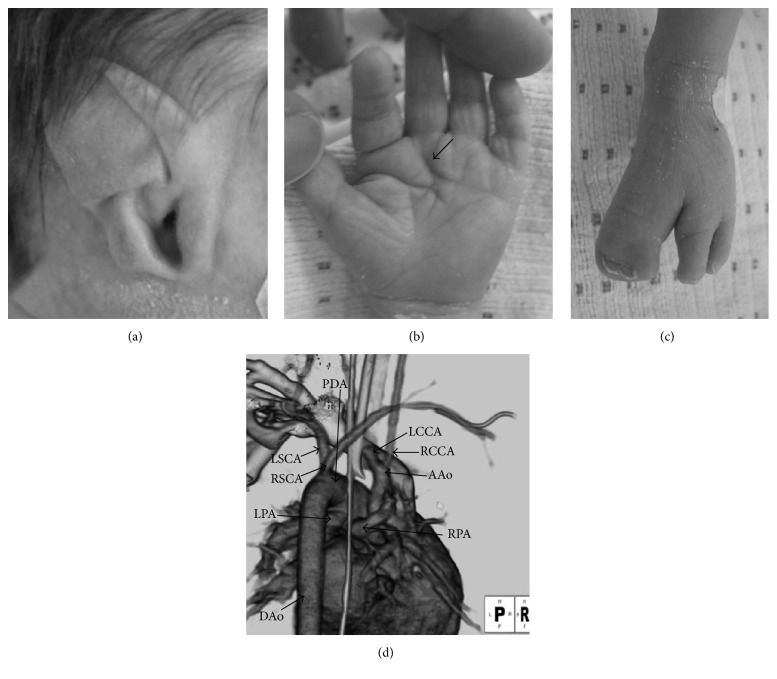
Dysmorphic features and cardiovascular malformations in Case 1. (a) “CHARGE ear.” (b) Hockey-stick palmar crease (arrow). (c) Cleft foot. (d) 3D-CT angiography showed truncus arteriosus type A4, interrupted aortic arch type B, and aberrant origin of the right subclavian artery. AAo, ascending aorta; DAo, descending aorta; LCCA, left common carotid artery; LPA, left pulmonary artery; LSCA, left subclavian artery; PDA, patent ductus arteriosus; RCCA, right common carotid artery; RPA, right pulmonary artery; RSCA, right subclavian artery.
